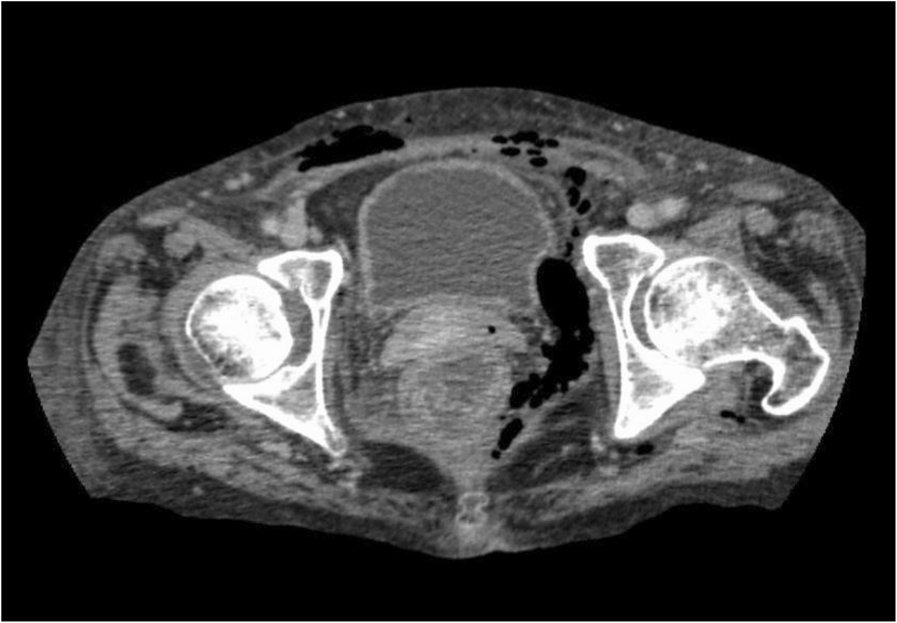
Computed tomography image of the lower-abdominal area before the surgery
